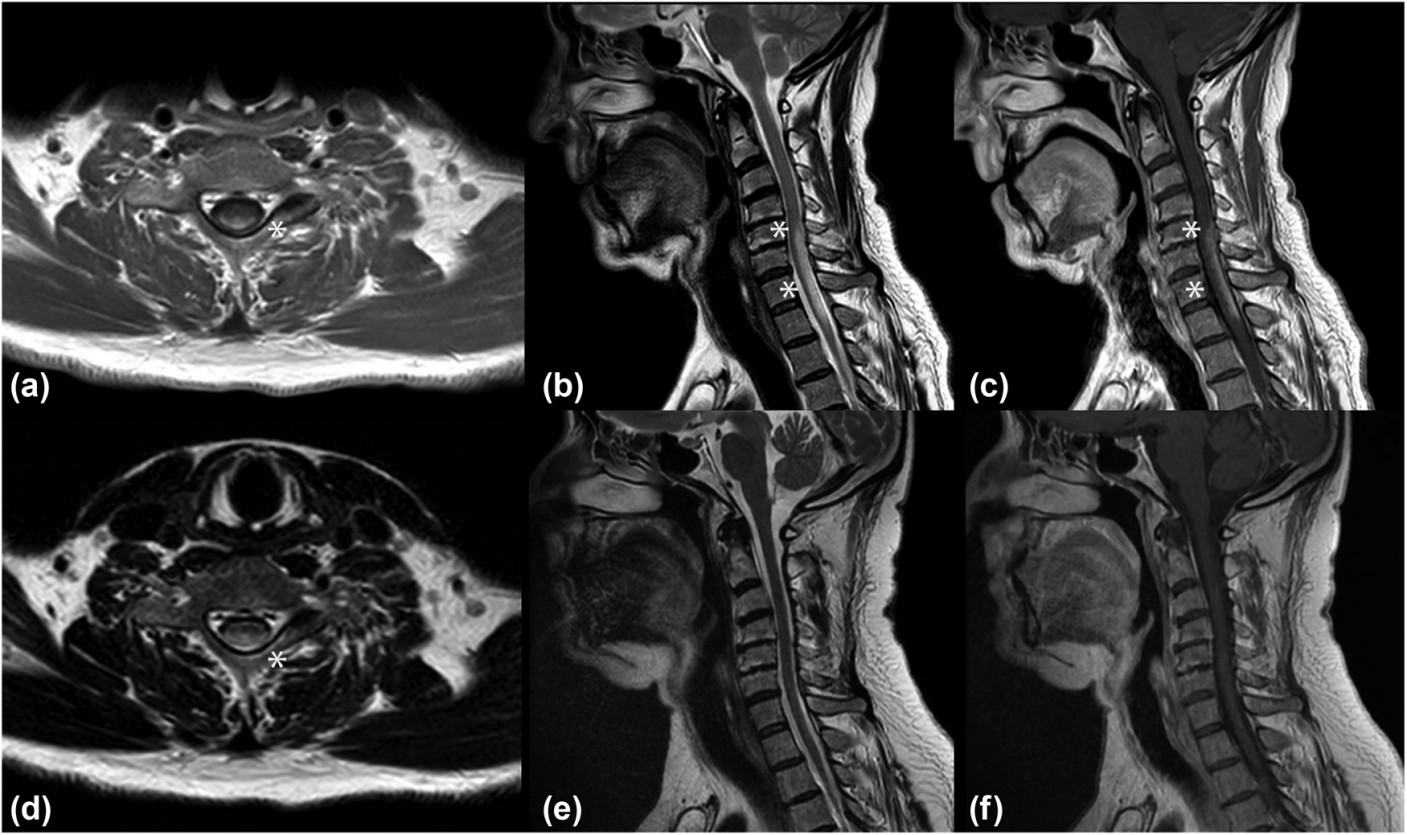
(a–d) Spinal MRI at clinical onset. (b) Sagittal T2-weighted image showing the hyperintense lesion extending from C5 to D2 (asterisks); (c) Sagittal T1 post-contrast sequence showing posterior subpial contrast enhancement (asterisks). Axial T1 post-contrast (a) and t2-weighted (d) sequences at symptoms’ onset showing the “trident sign” (asterisk). (e and f) spinal MRI 6 months after treatment. Sagittal T2-weighted (e) and T1 post-contrast (f) sequences showing an almost complete disappearance of the spinal lesion.
